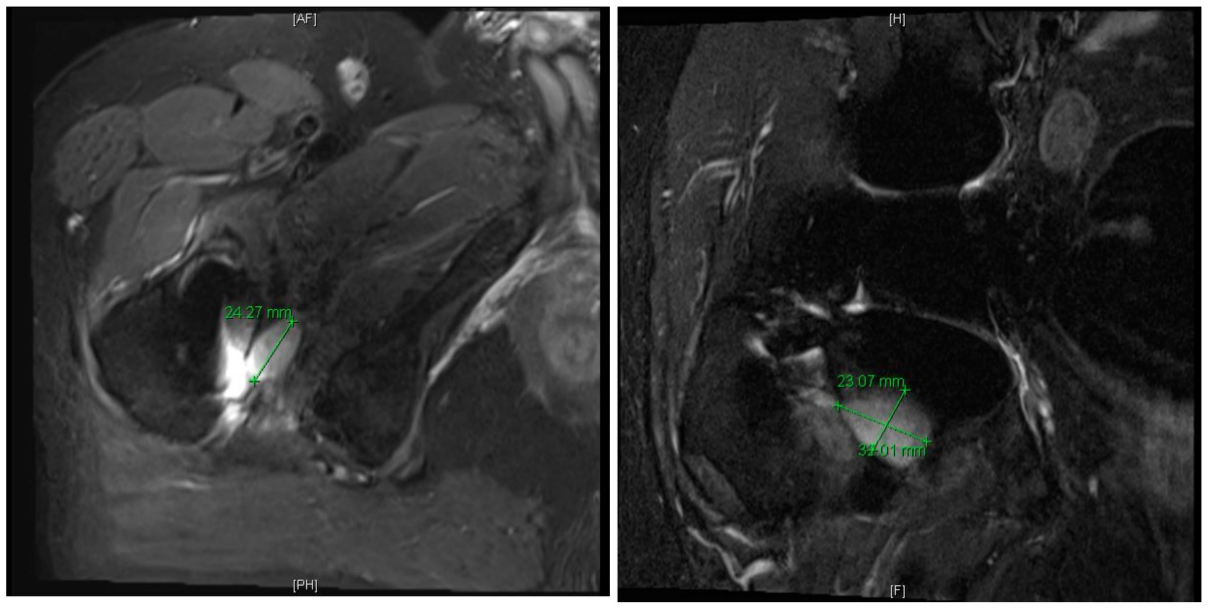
MRI right hip with findings of pseudotumor.
